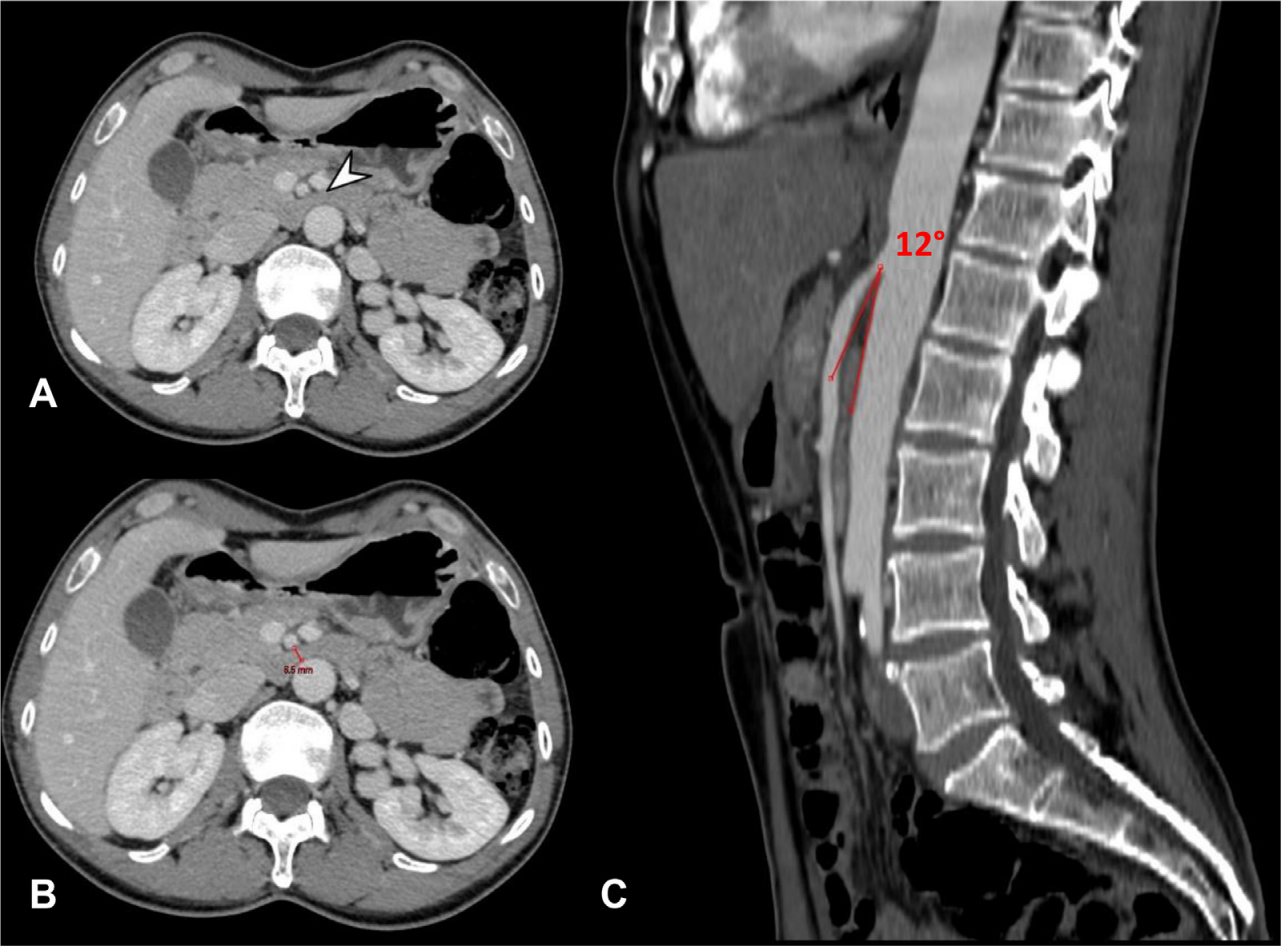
(A-C) Superior mesenteric artery syndrome. The SMA syndrome (also known as Wilkie's syndrome) is diagnosed radiologically when, in the presence of symptoms with the characteristics of a high intestinal occlusion, the presence of an angle between the SMA and the aorta <22° or when the aortomesenteric distance is <8 mm. Axial CE-CT images of our case (A,B), obtained in portal venous phase, demonstrate the presence of duodenal compression (arrowhead in A) and a maximum aortomesenteric distance of 6.5 mm (red segment in B). In addition, sagittal CE-CT image (C) shows the presence of an aortomesenteric angle of approximately 12° (red angle in C).
